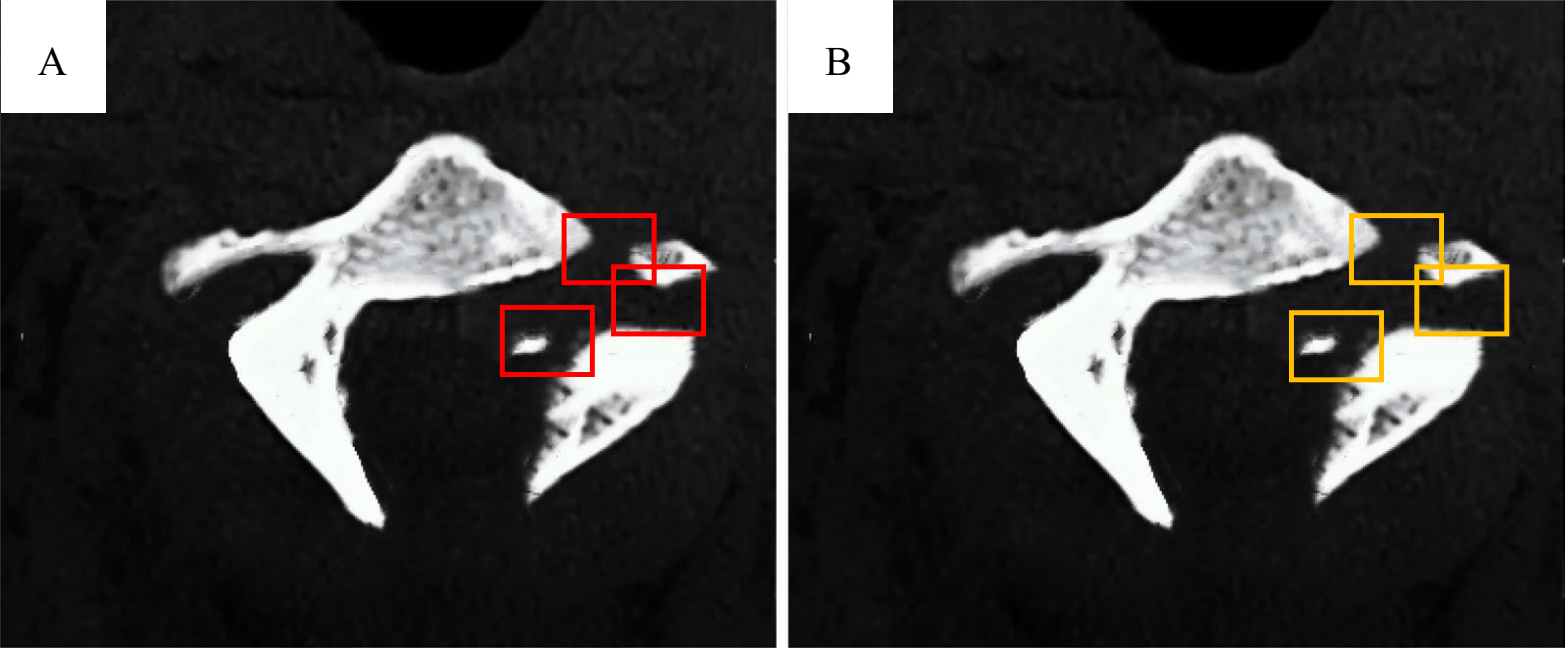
Effects of computer CT intelligent detection. A Artificial annotation of fractured sites in CT images. B Fractured sites in CT images annotated by DCNN model
